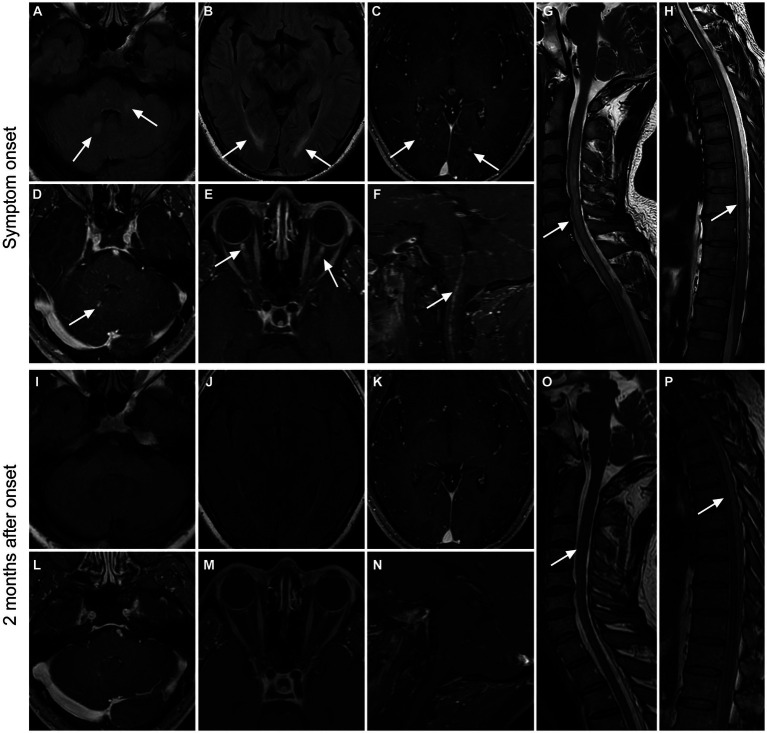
Imaging findings in a patient with GFAP. A 36-year-old male presented with bilateral lower limb weakness and numbness, accompanied by unsteady gait, with a subacute onset. After treatment with glucocorticoids, intravenous immunoglobulin, and tacrolimus, there was an improvement in symptoms, and a follow-up examination after 2 months showed a mRS score of 1. Initial MRI examination during the onset of the disease (A–H) revealed high T2WI signal in the cerebellar hemisphere and brainstem (A), as well as in the periventricular white matter surrounding the bilateral lateral ventricles (B). Additionally, there were punctate enhancements observed in the periventricular regions surrounding the bilateral lateral ventricles (C, arrows), as well as in the cerebellar hemisphere and brainstem (D, arrow). Furthermore, there was prominent enhancement in the anterior-middle portion of the bilateral optic nerves (E) and linear enhancement in the central canal of the medulla oblongata and cervical spinal cord (F). The cervical spinal cord (G), thoracic cord, and conus medullaris (H) had hyperintensity on T2WI throughout the entire segments. Follow-up MRI examination at 2 months after onset (I–P) showed the disappearance of T2WI hyperintensity and enhanced lesions in the brain parenchyma (I–L). The enhancements in the optic nerves and medulla oblongata-cervical cord central aqueduct disappeared (M,N). Most of the T2-weighted hyperintensity lesions in the spinal cord were resolved (O,P).
